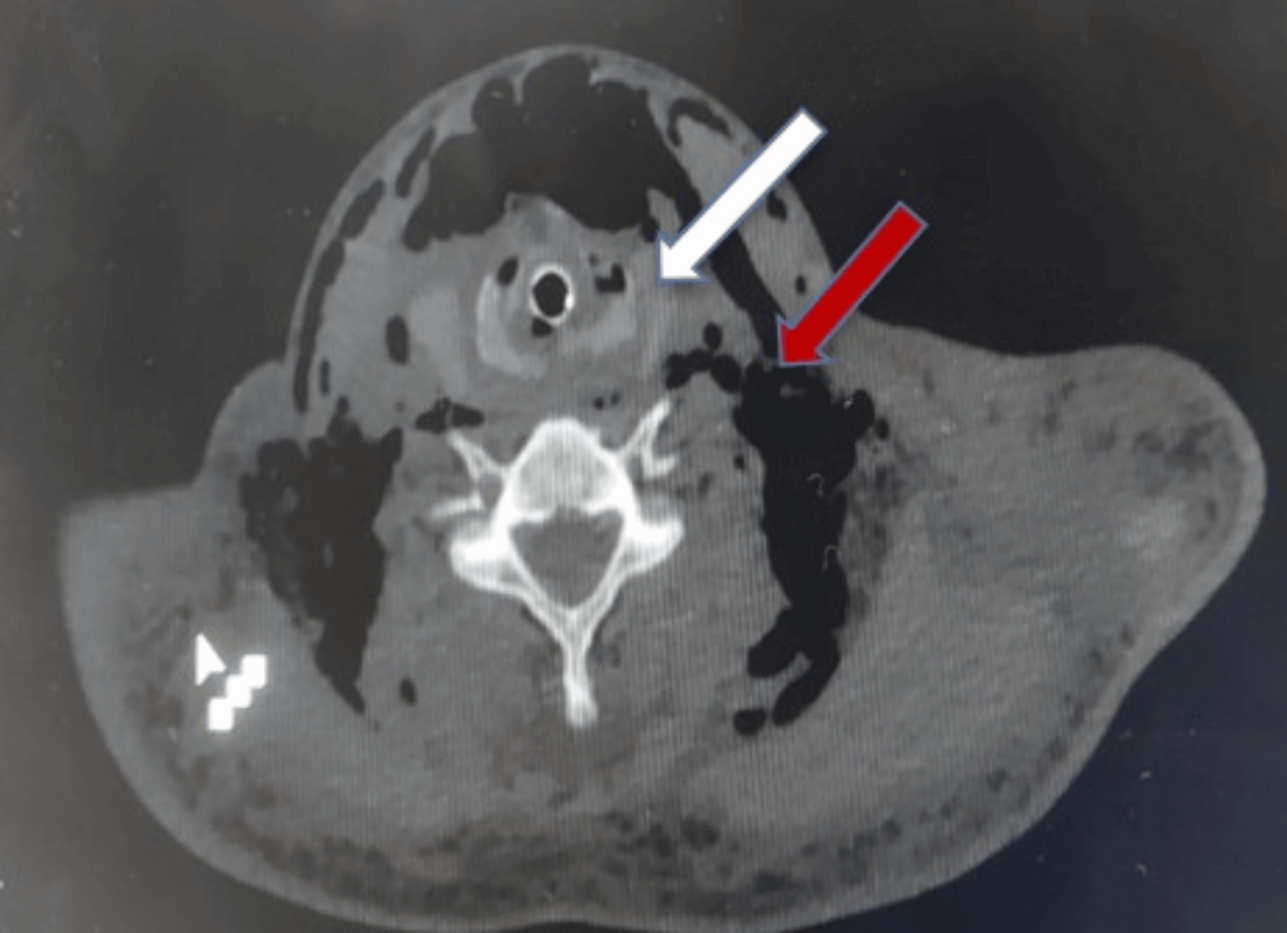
CT scan showing subcutaneous emphysema around the neck (red arrow) with distortion of laryngotracheal framework (white arrow)
